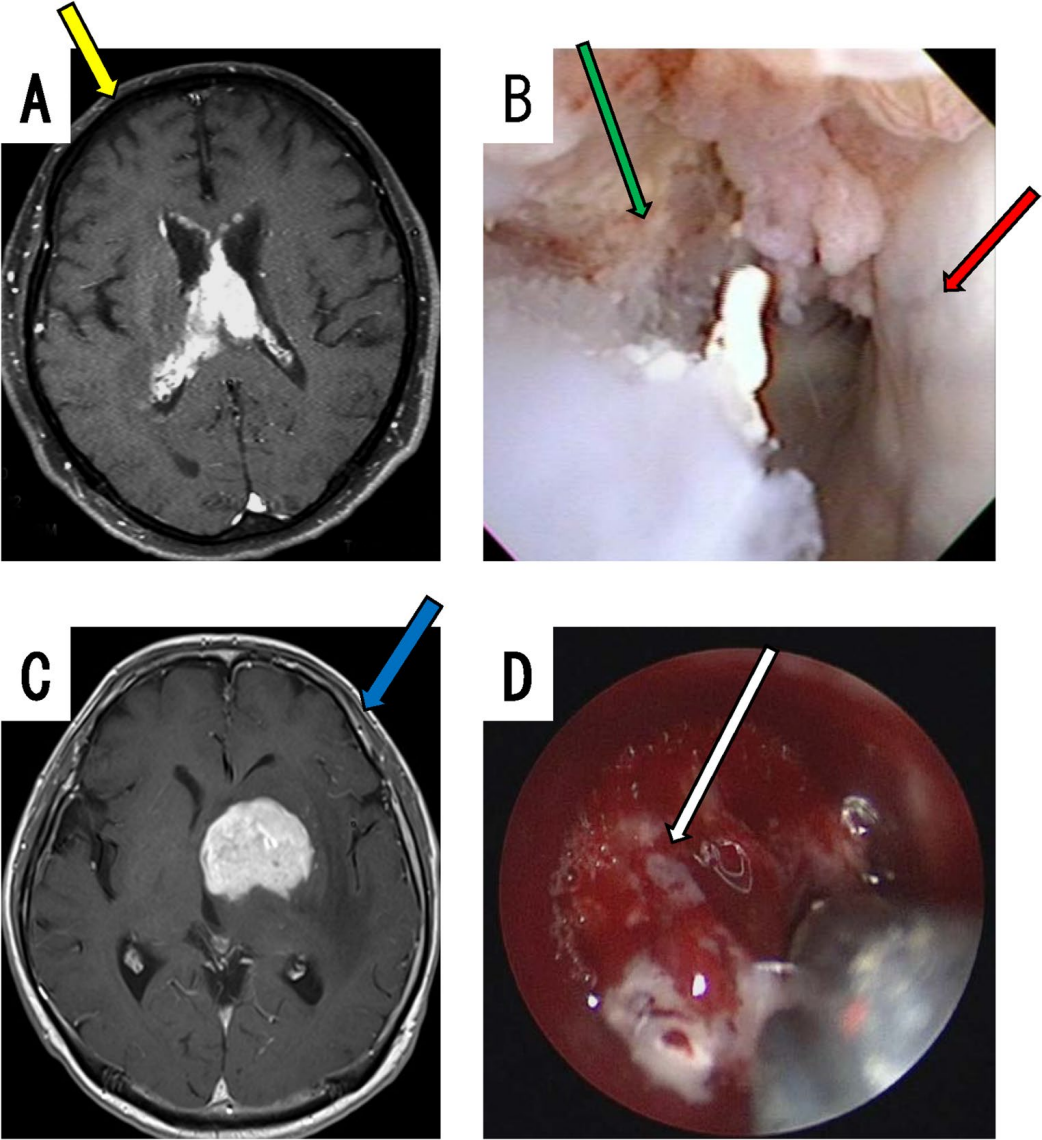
Two representative cases of neuroendoscopic biopsy. Case A: MRI displayed enhanced lesions in the septum pellucidum within the ventricles, and the lateral ventricular walls were also partly contrast-enhanced (A). The lesion was approached from the right anterior horn of the lateral ventricle (A, yellow arrow: direction of sheath insertion). An obvious lesion was found within the ventricle (B, green arrow), and the ventricular wall were reduced in transparency (B, red arrow). Case B: MRI displayed a lesion in the left caudate head (C). The lesion was approached using a ridged scope (C, blue arrow: direction of sheath insertion). Endoscopy demonstrated that the tumor was dark red (D, white arrow)
